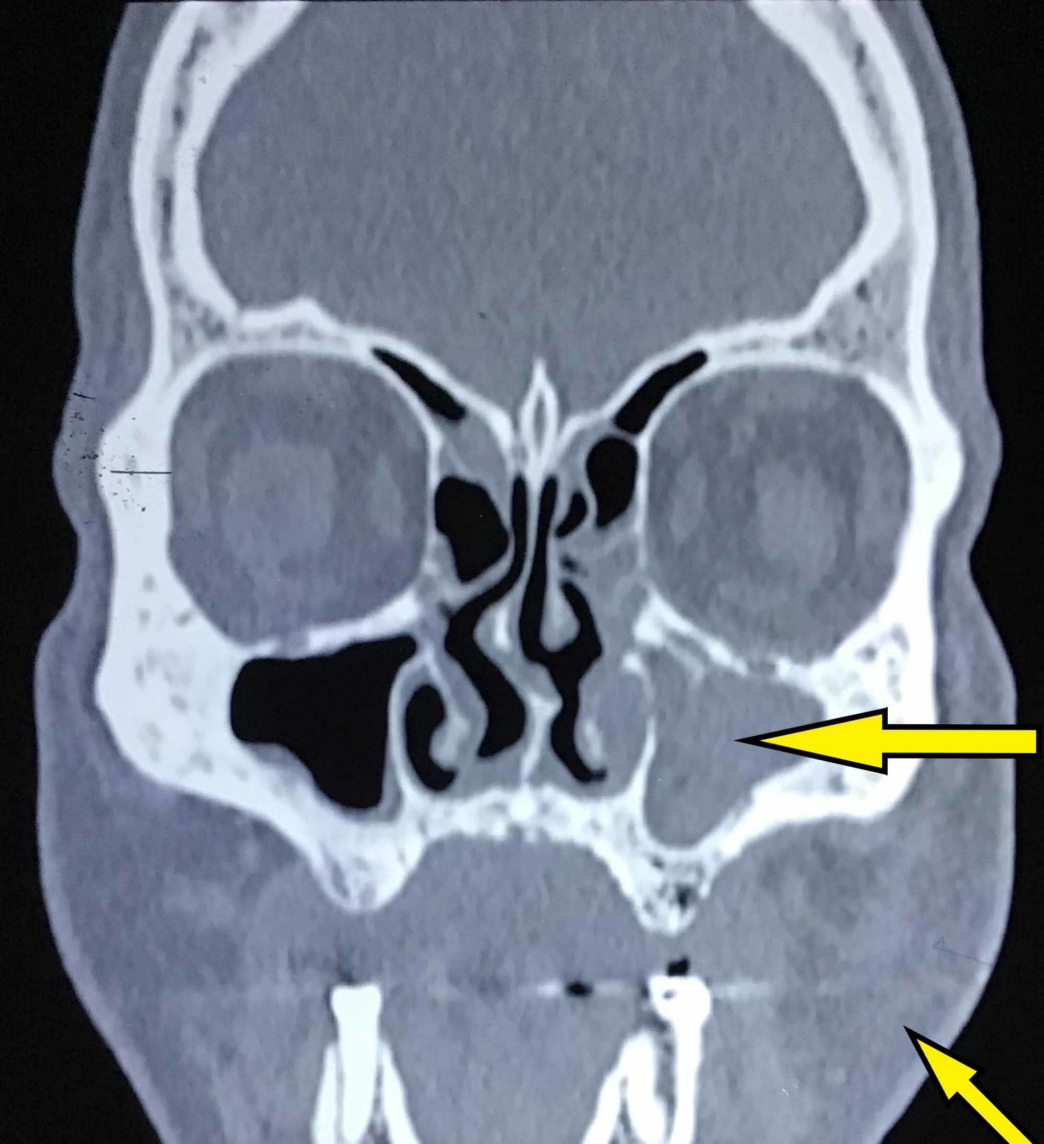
Preoperative facial CT showing facial swelling on the left side of face and obliteration of the left maxillary sinus.
